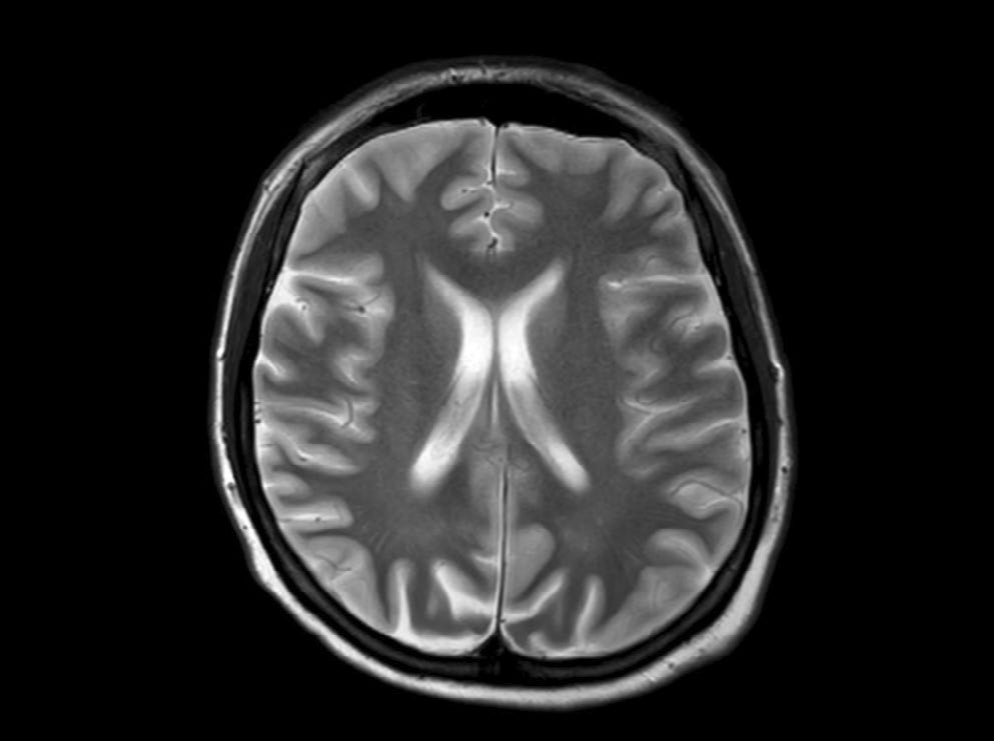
FIGURE 2 FLAIR hyperintensities in the bilateral cortical surfaces of frontoparietooccipital lobes
